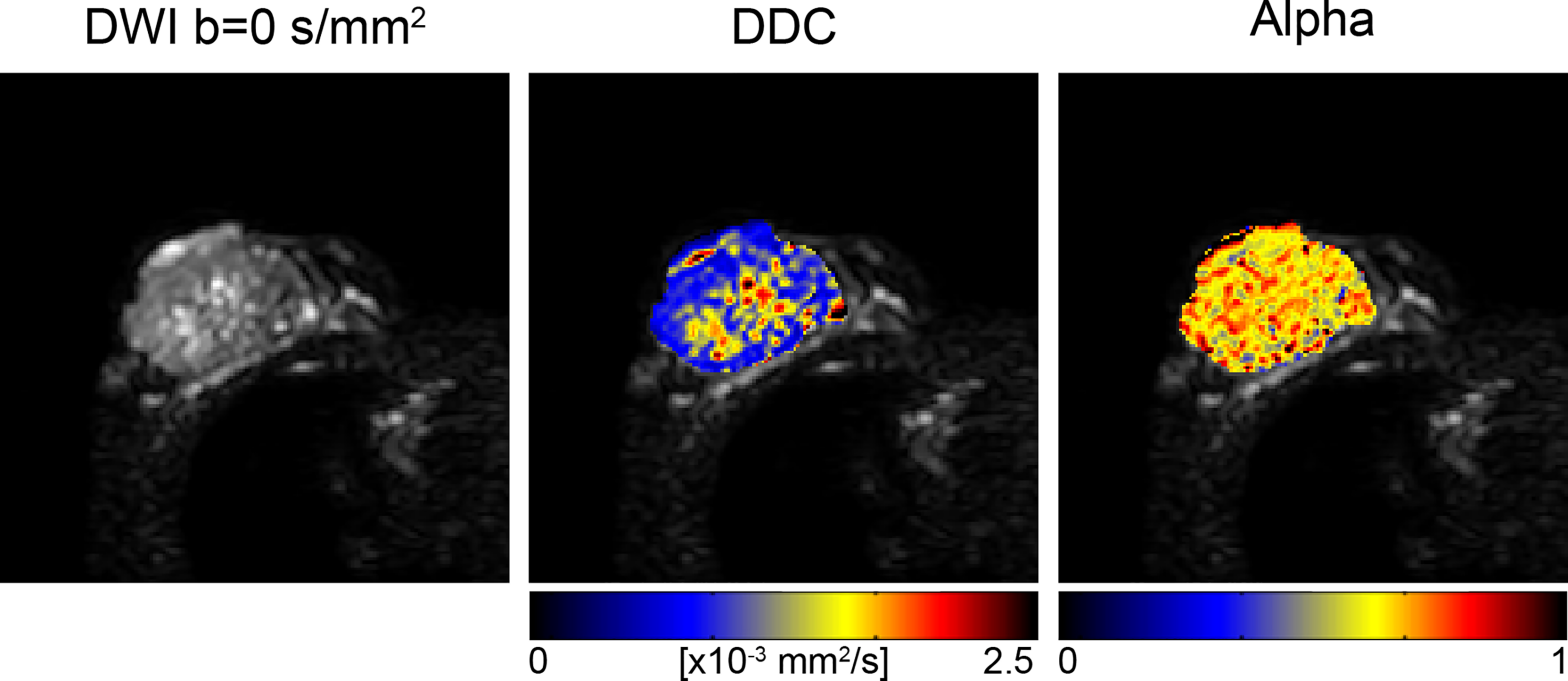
Stretched exponential modeling with b-values of 0, 500, 1000, 1500 and 2000 s/mm^2^ in a 73-year-old patient with invasive ductal carcinoma. Distributed diffusion coefficient (DDC) and alpha maps are overlaid on DWI b=0 s/mm^2^ images, acquired at 3T (56). (Courtesy of Shiteng Suo, PhD, and Jia Hua, MD, Department of Radiology, Ren Ji Hospital, School of Medicine, Shanghai Jiao Tong University, Shanghai, China).
